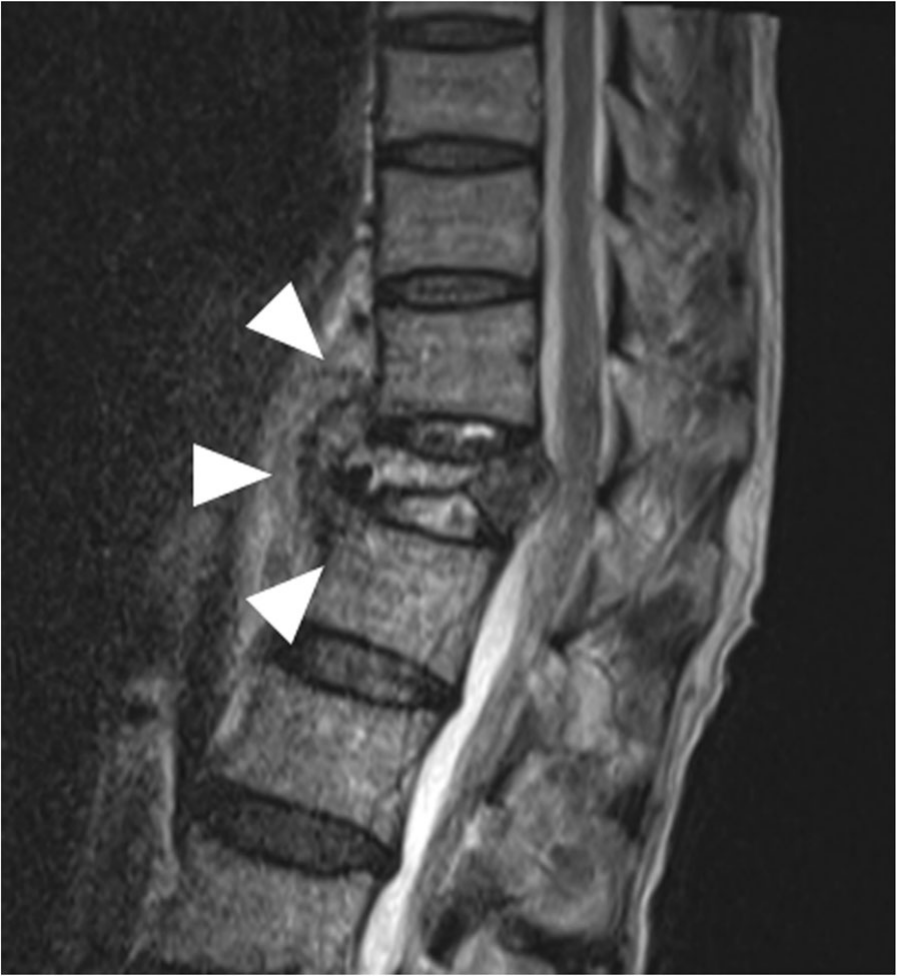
Lumbar magnetic resonance imaging (MRI) on admission. MRI revealed a compression fracture of the 12th thoracic vertebral body and hematoma anterior to the vertebral body (white arrowheads)
